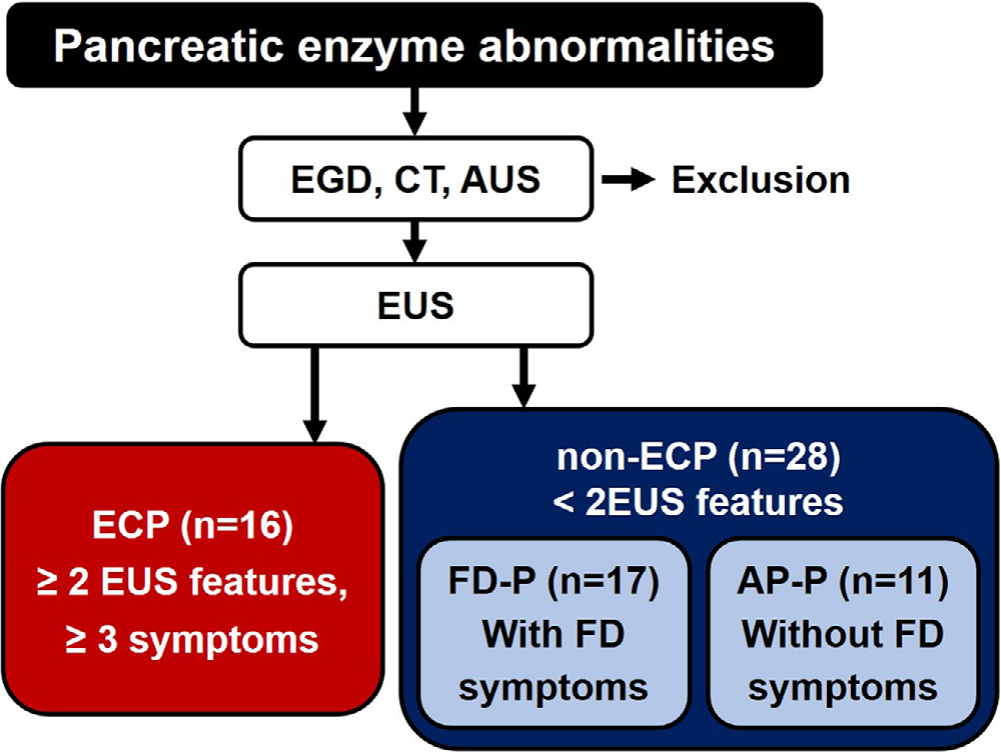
The protocol of the study. ECP; early chronic pancreatitis, FD; functional dyspepsia, FD‐P; functional dyspepsia with pancreatic enzyme abnormalities, AP‐P; asymptomatic patients with pancreatic enzyme abnormalities, EGD; esophagogastroduodenoscopy, CT; computed tomography, AUS; abdominal ultrasonography, EUS; endoscopic ultrasonography.
